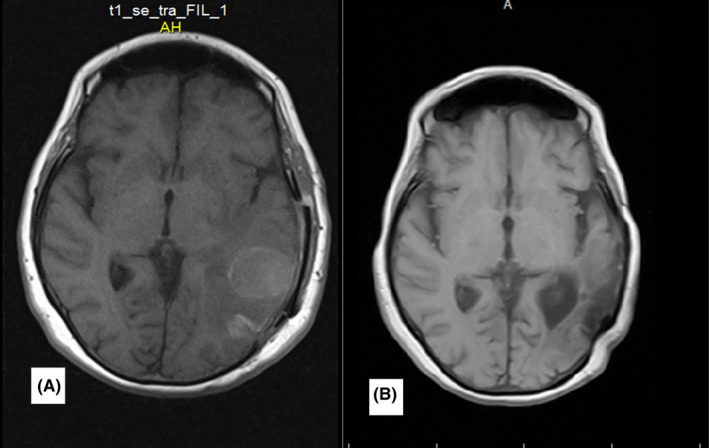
Case number 2. (A) brain MRI before treatment. Post‐contrast axial cut. A faint heterogeneous mass with mass effect in the left parietal lobe. (B) brain MRI after 30 months follow‐up. Axial brain MRI without contrast. Gliomalacia in the left parietal lobe
